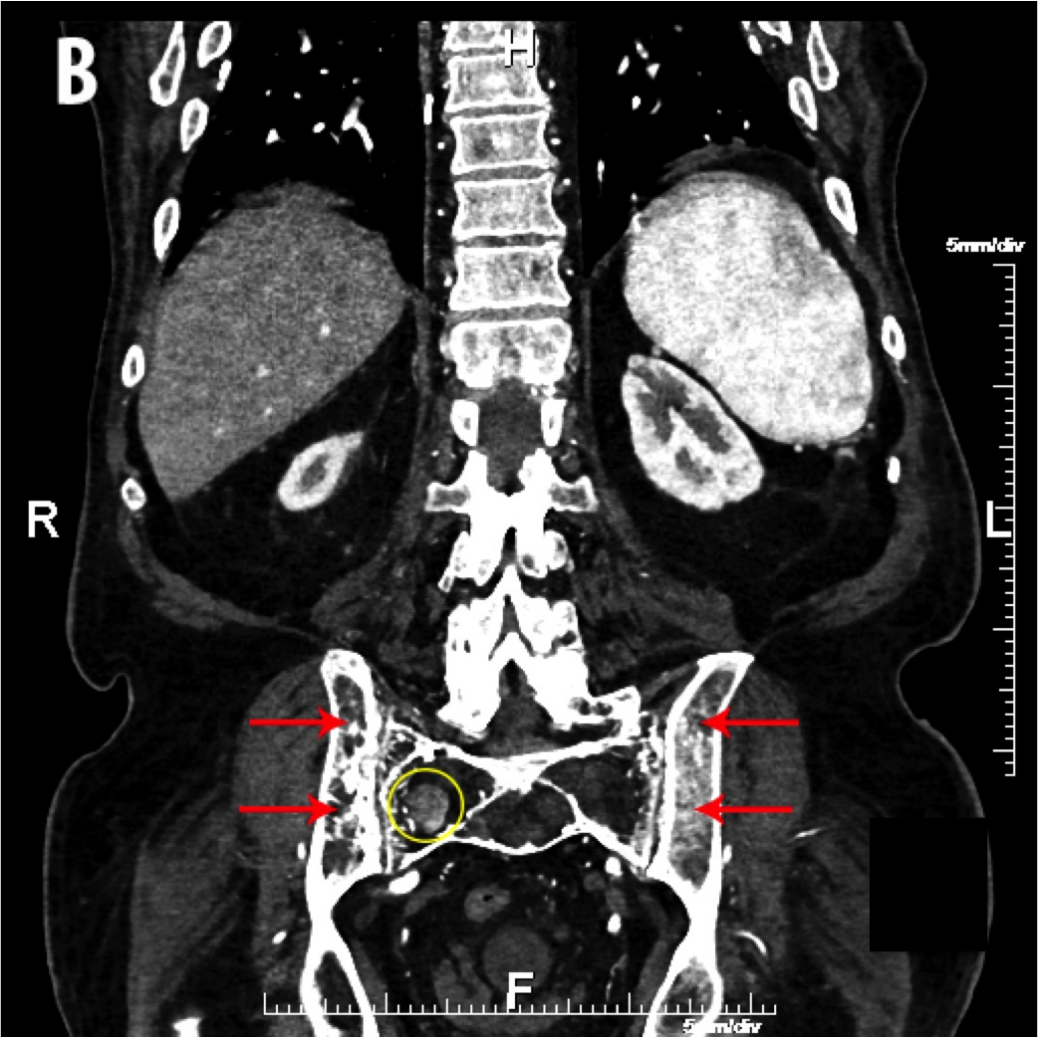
A Coronal CT of the Abdomen and Pelvis Indicating Metastatic Renal Cell Carcinoma in Pagetic Bone A coronal contrast-enhanced CT through the abdomen and pelvis: concomitant Paget’s disease (red arrows) and metastatic renal cell carcinoma (yellow circle) in the right sacrum.
